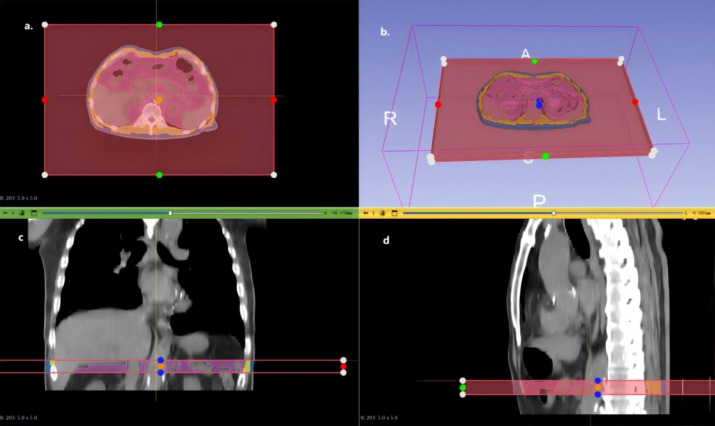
The measurement of body composition on chest CT in a 77-year-old female patient with viral pneumonia. (A) T12 costovertebral joints and (B) all vertebra. In the axial image localisation, the region of interest (ROI) of T12 corresponds to (C) the coronal and (D) sagittal reconstruction images. The blue area represents subcutaneous fat, the purple area represents visceral fat, and the yellow area represents the muscle.
